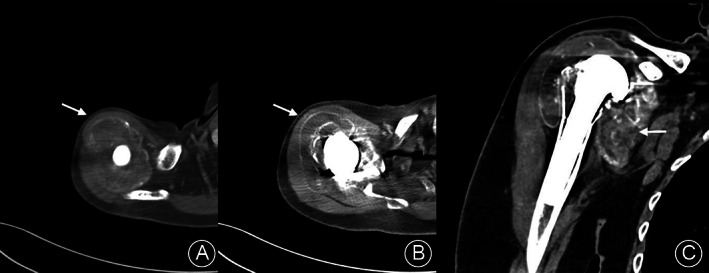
Contrast‐enhanced computed tomography scans showed rim‐enhanced and non‐enhanced fluid collection around the sub‐acromial bursa (A, arrow), subdeltoid (B, arrow), and glenohumeral joint (C, arrow).
